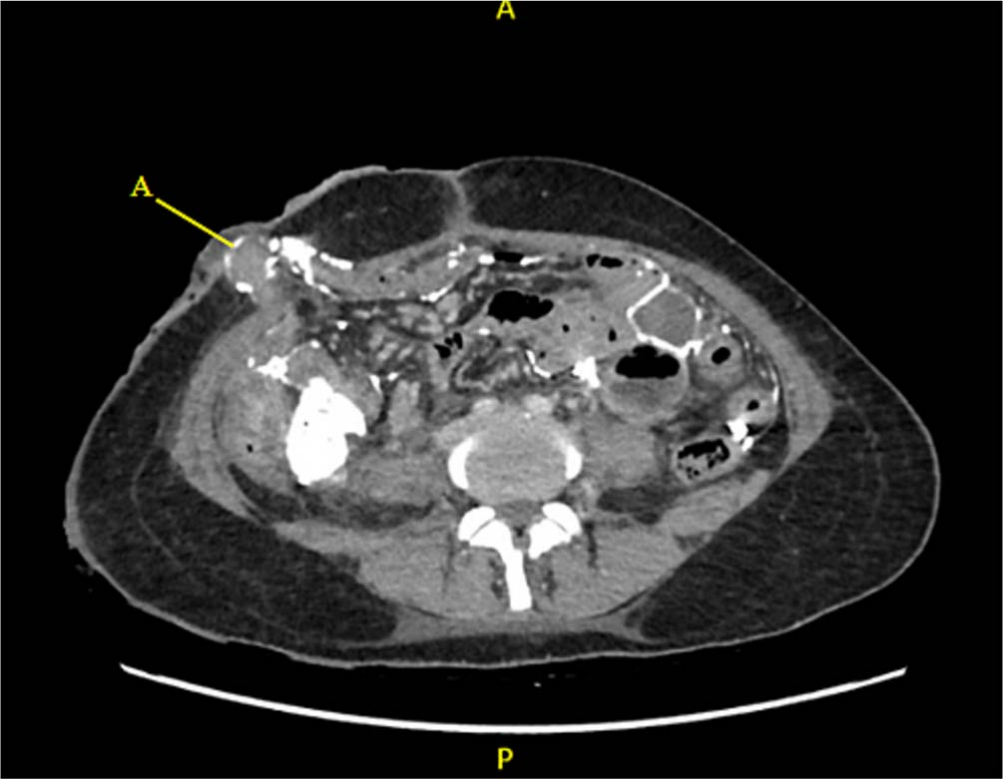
CT contrast abdomen and pelvis showing spill of barium outlining the peritoneal cavity. Stoma at the right side of the abdomen (A).
